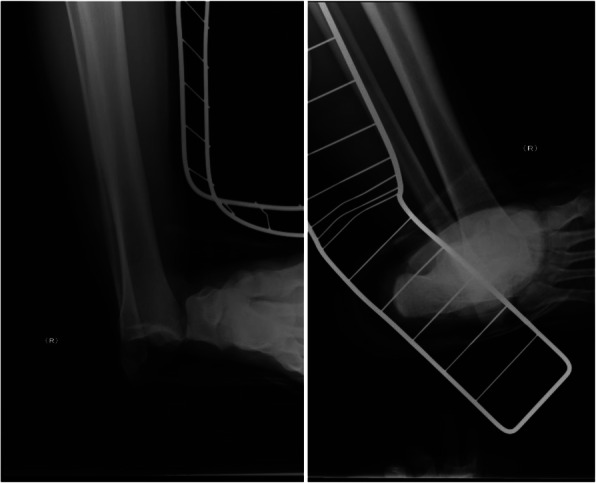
Plain x-ray image obtained at the first visit to our hospital. The tibiotalar joint was found to be dislocated in the medial direction without any fractures
